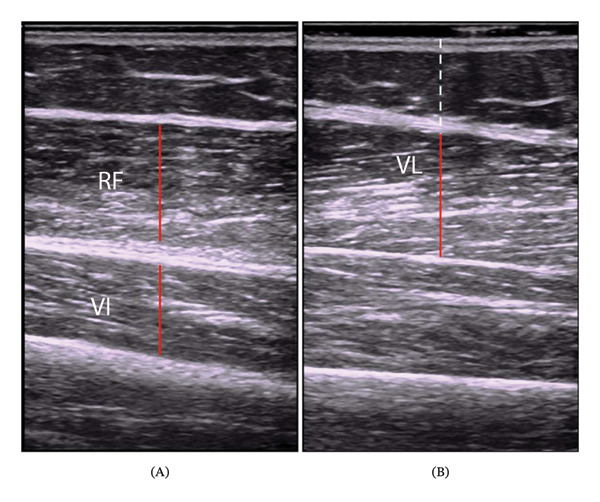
Ultrasonography images of (A) rectus femoris (RF) and vastus intermedius (VI) and (B) vastus lateralis (VL) muscles. Red lines indicate measurements of muscle thickness, while white dashed line indicates measurements of subcutaneous tissue (including fat, skin, and aponeurosis).
